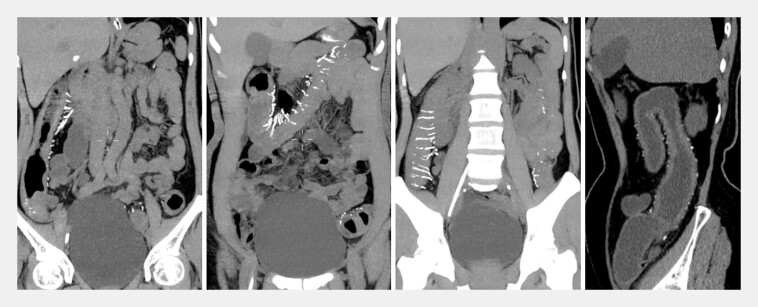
Noncontrast abdominal CT showing extensive calcification and uniform thickening of the colonic wall.
